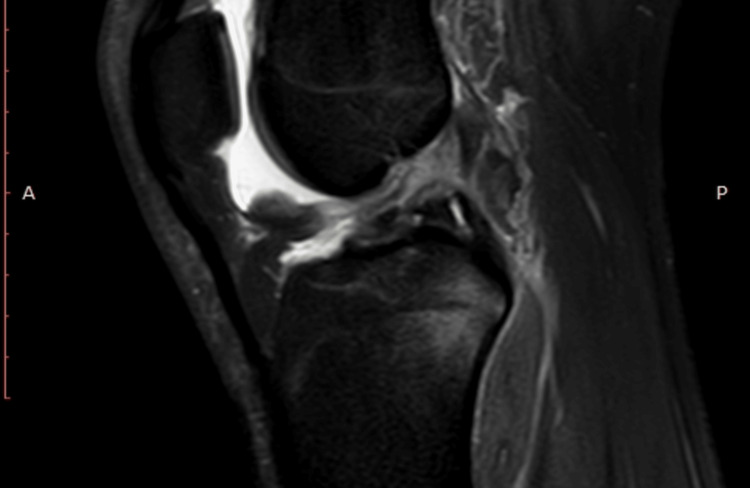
MRI showing anterior cruciate ligament (ACL) tear
